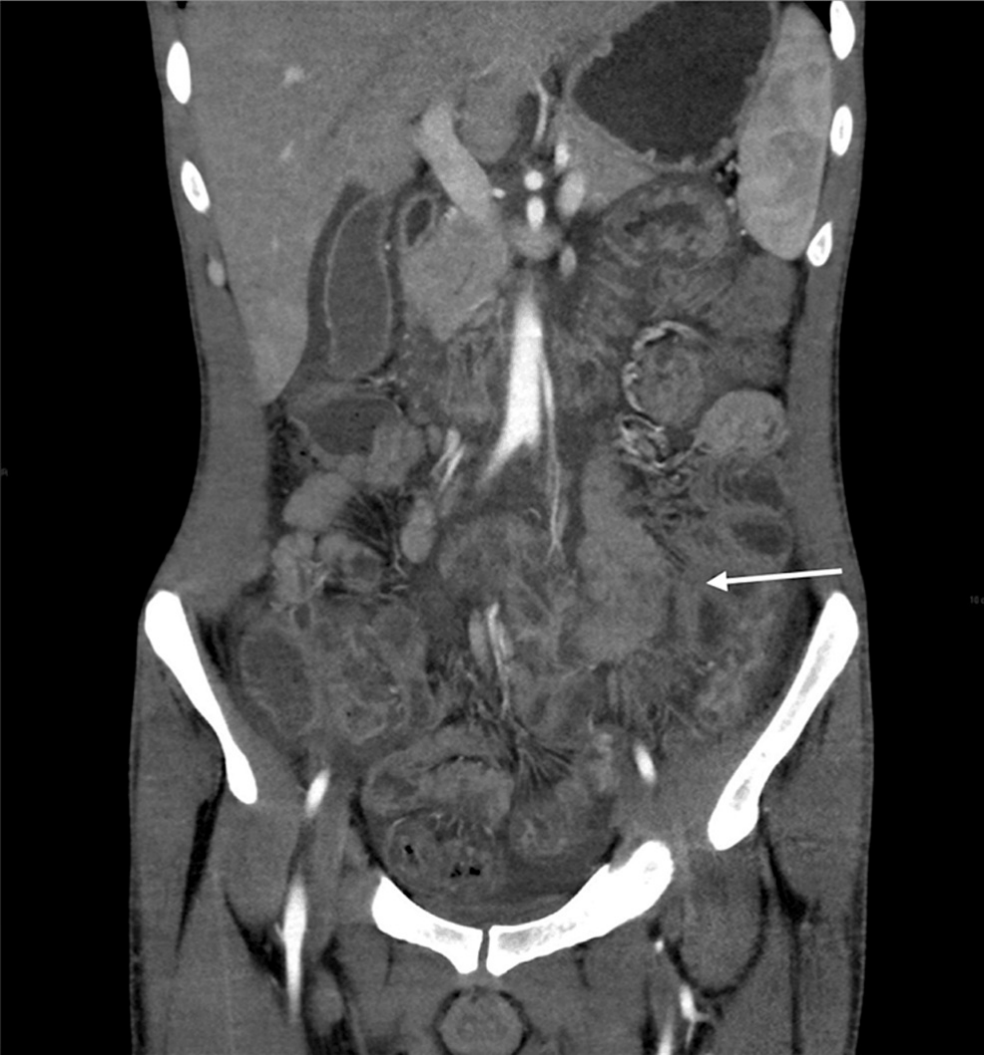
Abdominal CT showing intestinal wall thickening (white arrow)
